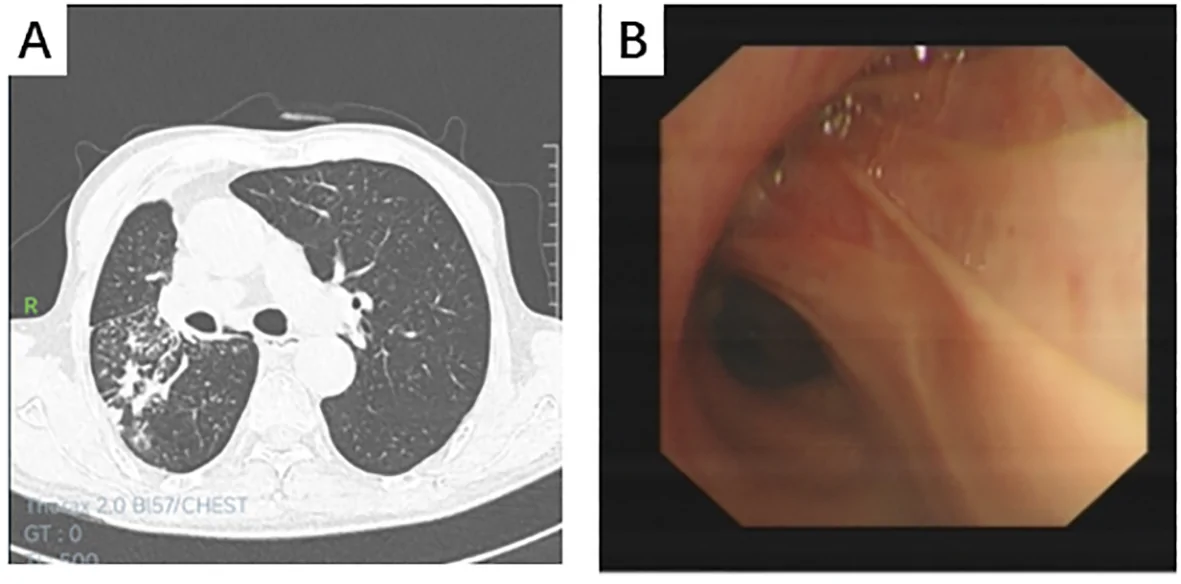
(A) Chest CT scan in July 2025, showed multiple bilateral pulmonary infiltrates and ground-glass opacities, accompanied by right pleural thickening. (B) Bronchoscopy revealed the stump of the right upper lobe bronchus; the mucosa of the remaining lobar and segmental bronchi was smooth with patent lumens. Bronchoalveolar lavage (BAL) was performed at the dorsal segment of the right lower lobe.
